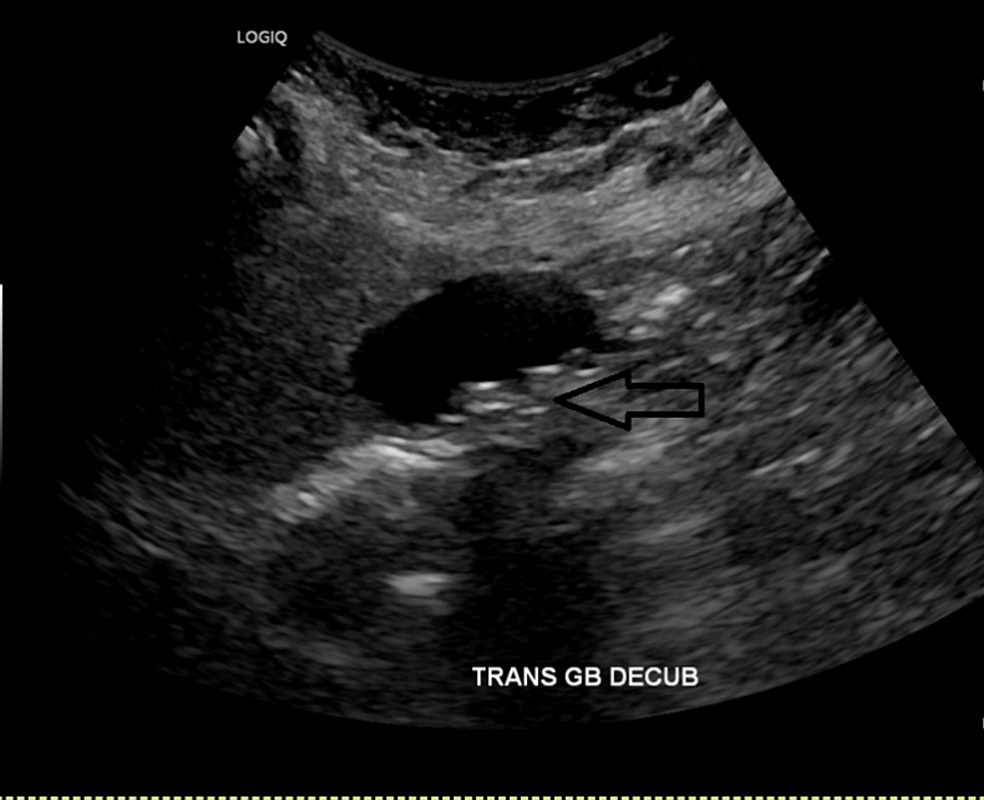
Abdominal ultrasound Abdominal ultrasound showing multiple subcentimetric gallstones without evidence on cholecystitis (arrow).
